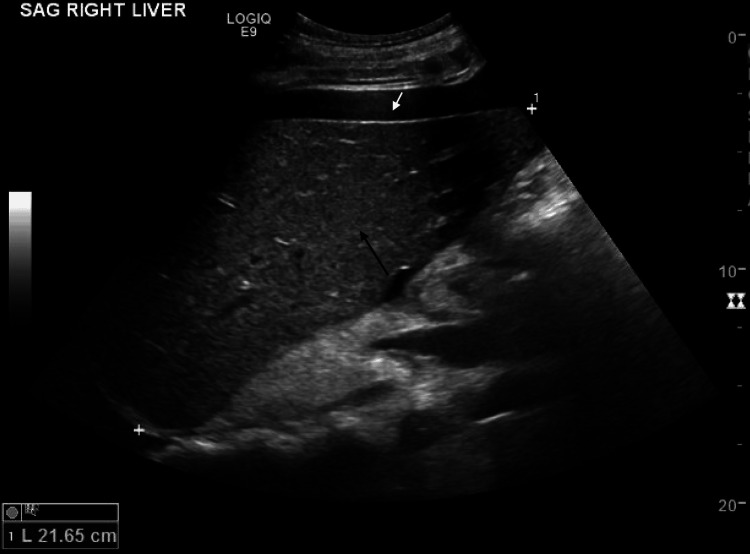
Ultrasound of the liver (sagittal) showing heterogeneous echogenicity (black arrow), ascites (white arrow), and hepatomegaly (cranio-caudal dimension 21.65 cm).
